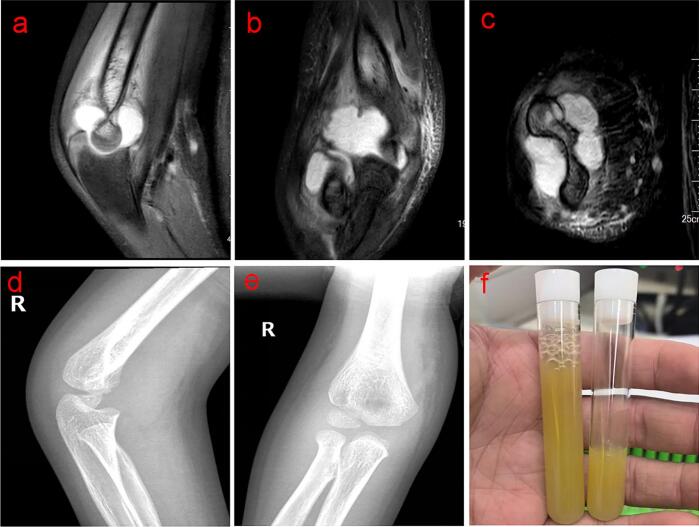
The preoperative status of the patient's elbow joint. a, b, c: MRI demonstrates joint effusion, synovial thickening and swelling; d, e: plain imaging shows no significant abnormalities; f: joint aspirated the cloudy synovial fluid.
